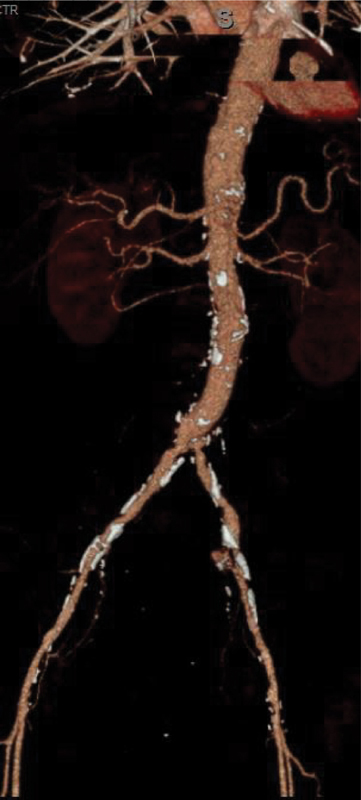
Three-month follow-up computed tomography angiography showing patency.
